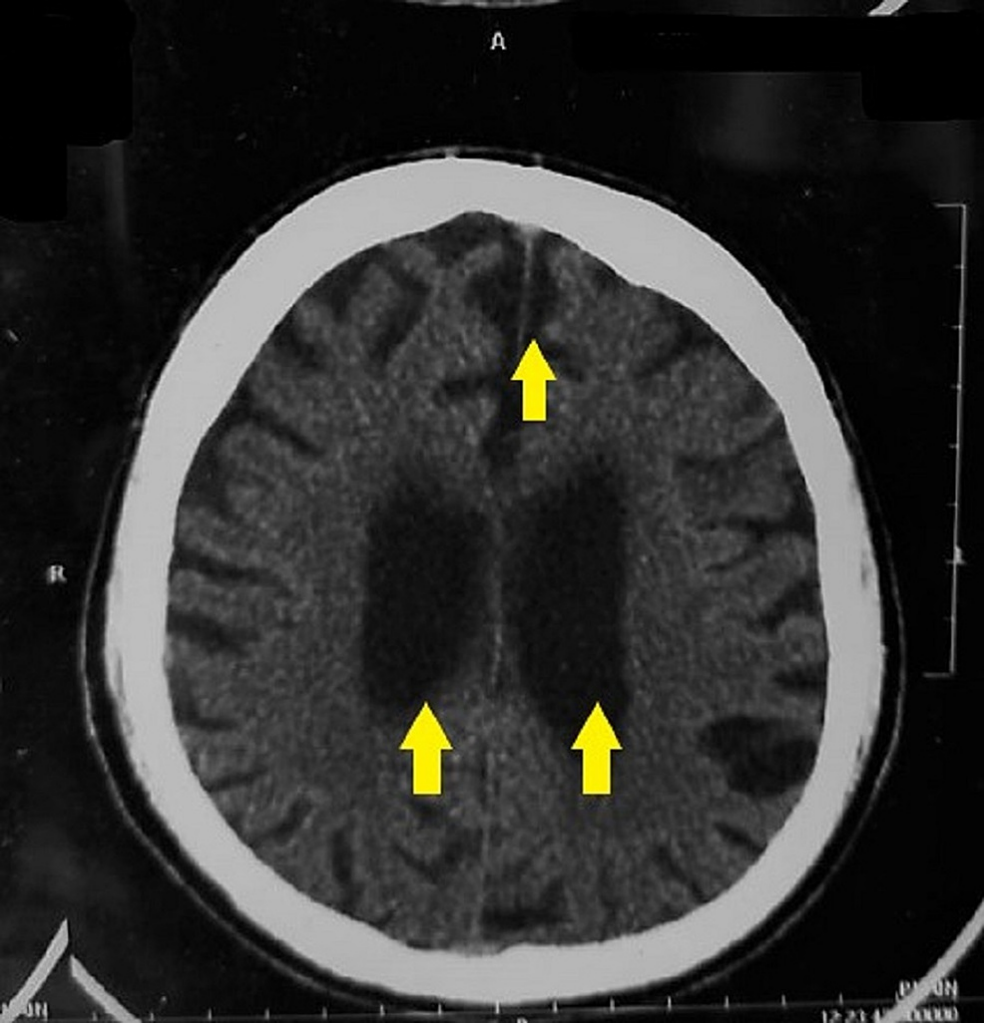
CT Brain showing bilateral cerebral atrophy in the form of enlarged cerebral ventricles and widened sulci (indicated by the colored arrows).
